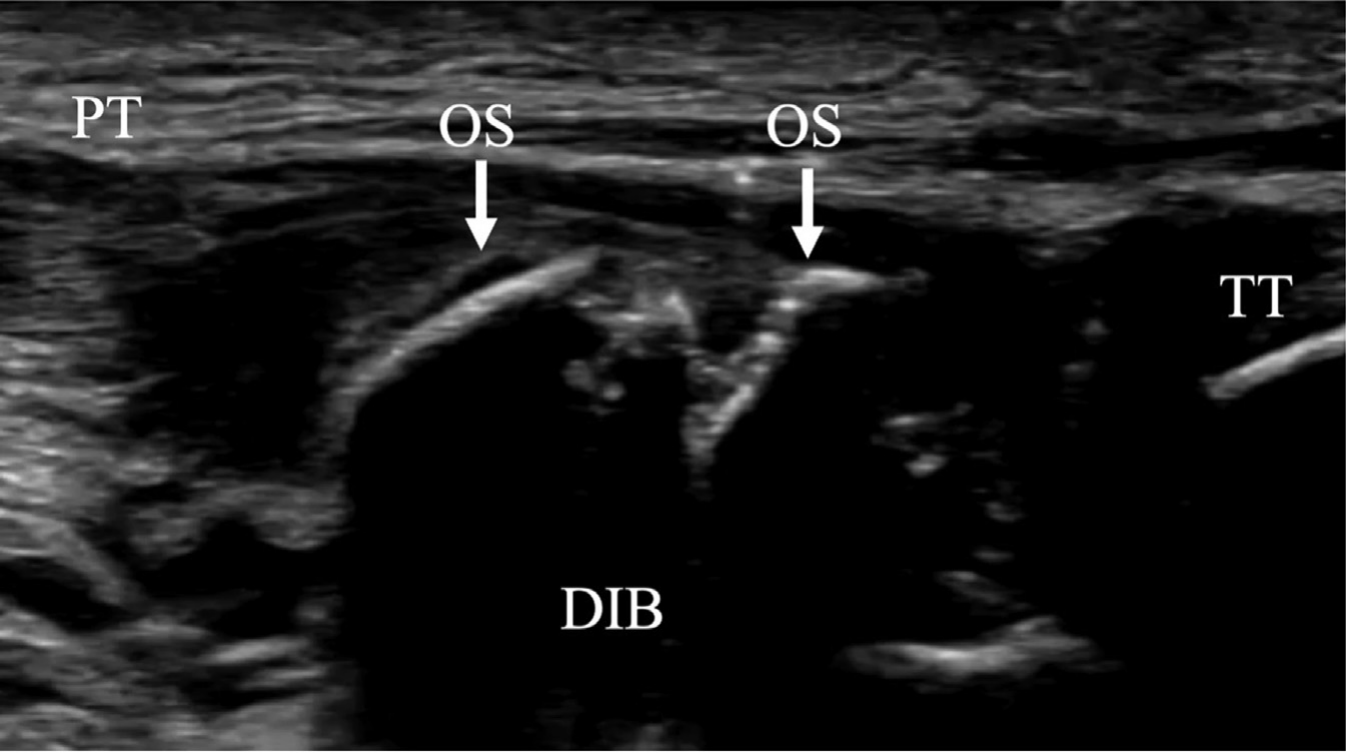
Preoperative ultrasonographic finding (long-axis image). Two ossicles are in the deep infrapatellar bursa. (DIB, deep infrapatellar bursa; OS, ossicle; PT, patellar tendon; TT, tibial tuberosity.)
